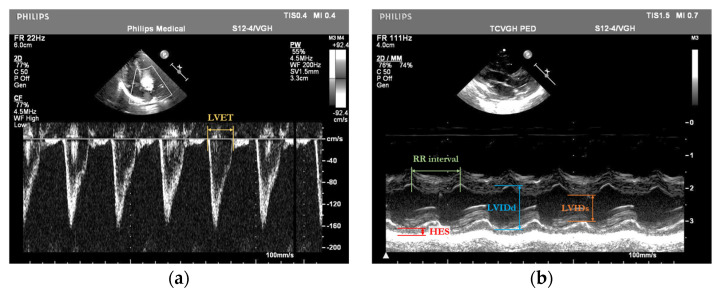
Annotation examples of (a) pulsed-wave Doppler-mode and (b) M-mode echocardiograms showing bounding boxes for LVET, LVIDd, LVIDs, HES, and RR interval.
